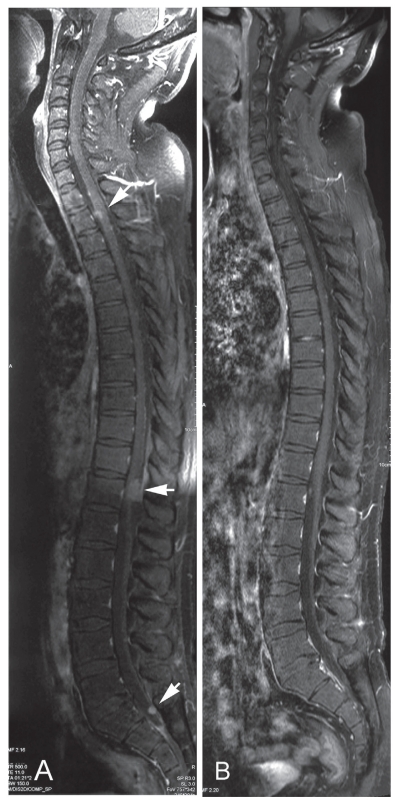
MRI of the spinal cord before and after salvage chemotherapy. 3A-Well-enhanced, multiple leptomeningeal metastases were detected at the 2nd and 12th thoracic cord levels, as well as at the 1st sacral cord level (Th2, Th12, and S1) (white arrows). 3B-Complete remission of the leptomeningeal metastases was demonstrated after salvage chemotherapy.
